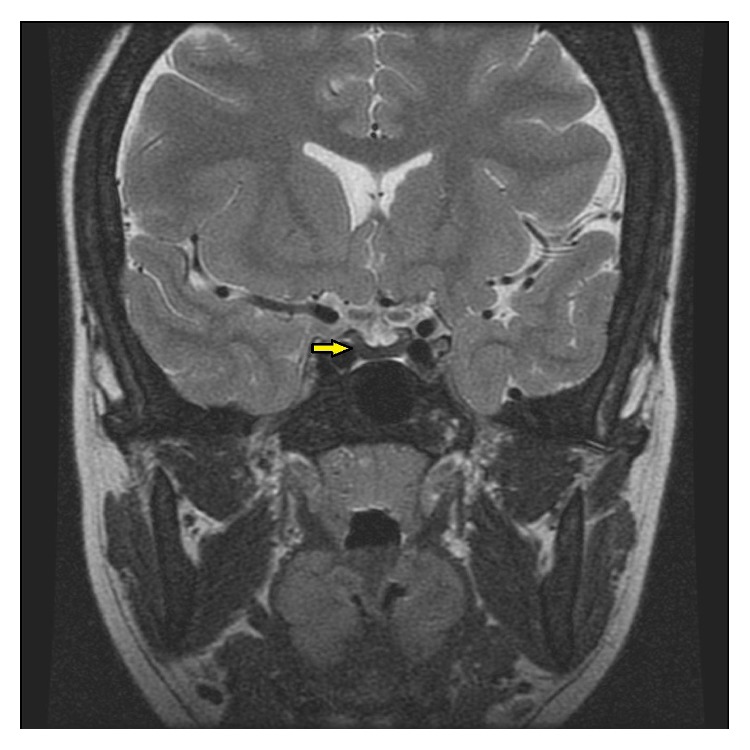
T2-weighted MRI showed decreased signal intensity of the pituitary gland, compatible with iron deposition.
